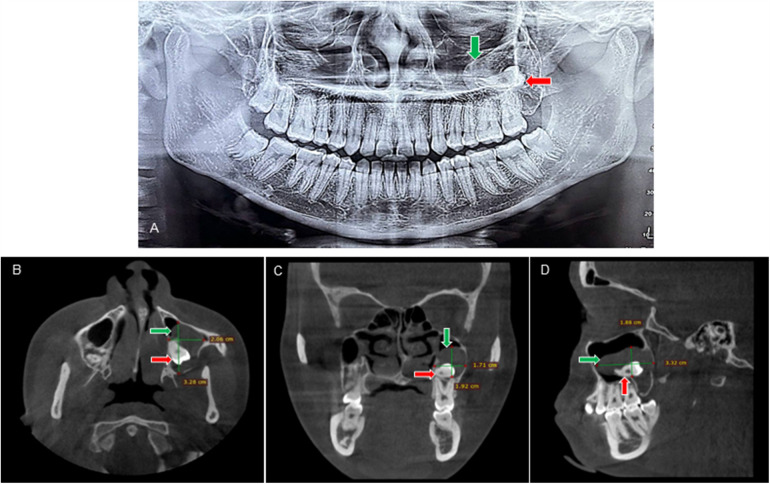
(A) preoperative orthopantomography: a radiolucent image with a central radiopaque focus compatible with an ectopic tooth in the left maxillary sinus is observed. Cone-beam computed tomography (CBCT): (B) axial, (C) coronal, and (D) sagittal views showing the ectopic left maxillary third molar associated with a well-defined hypodense image compatible with a cystic lesion. Red arrows indicate the ectopic left maxillary third molar, and green arrows indicate the margins of the associated cystic lesion.
